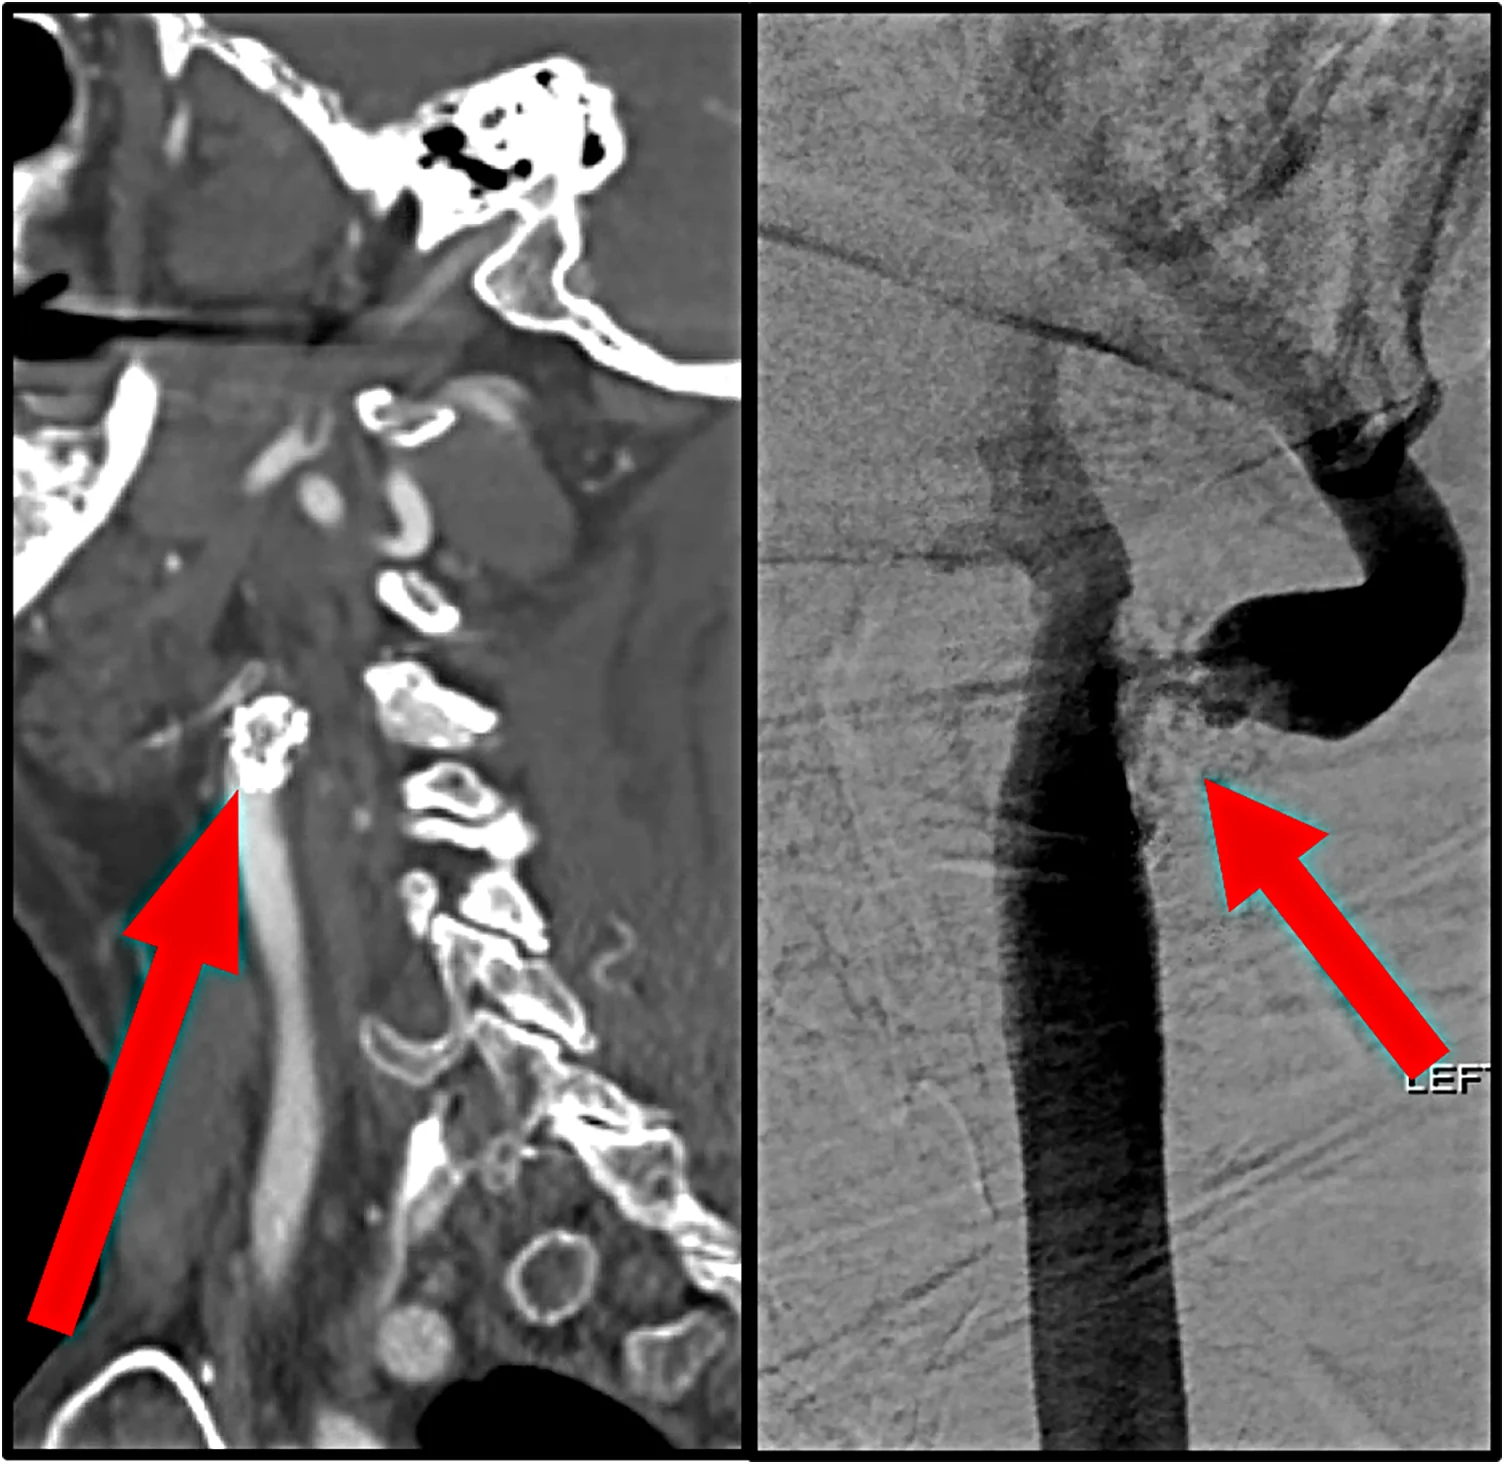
Pre-revascularization characterization of the left carotid bifurcation lesion. Left: Sagittal CTA of the neck demonstrating heavy near-circumferential calcification of the distal left common carotid army)/ and proximal internal carotid artery (arrow). Right: Selective left common carotid digital subtraction angiography confirming approximately 60% stenosis by visual NASCET estimate at the calcified carotid bifurcation lesion (arrow).
